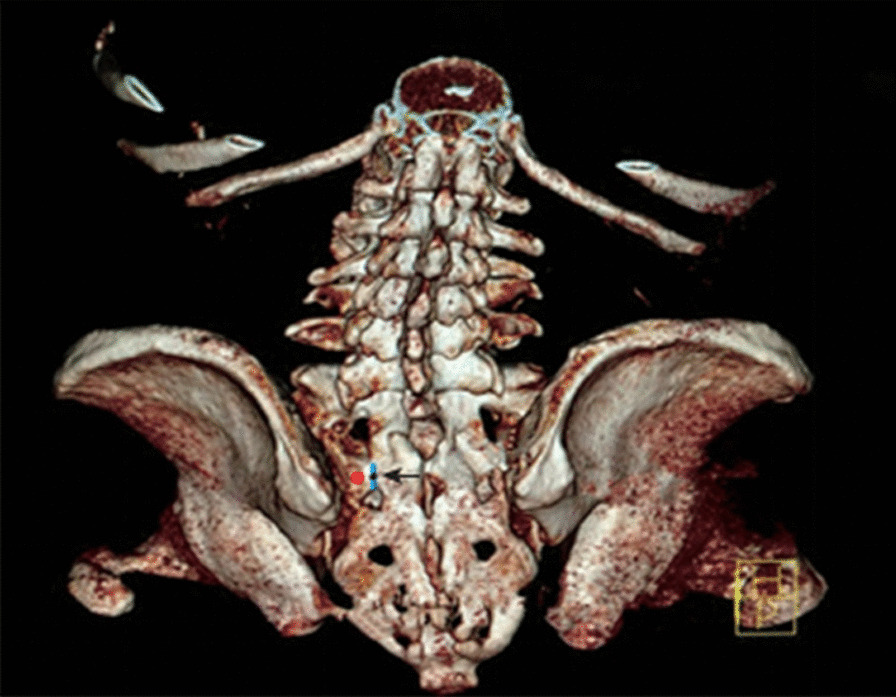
Three-dimensional CT reconstruction of the lumbosacral pelvis: red dot represents the insertion point/ starting point
